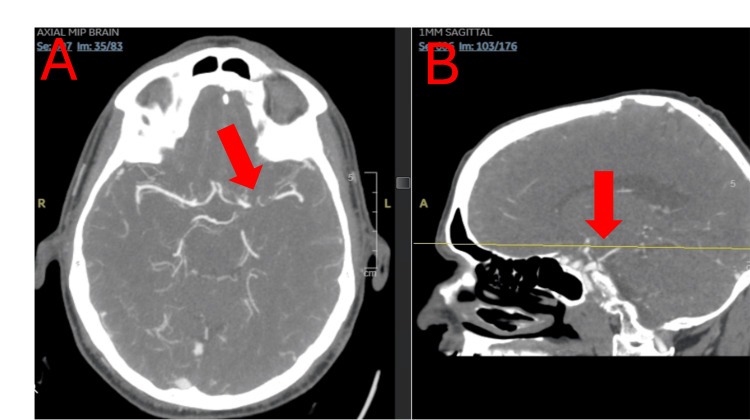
CT angiography without contrast displays bilateral anterior cerebral artery vasospasm (a) transverse; (b) sagittal views
